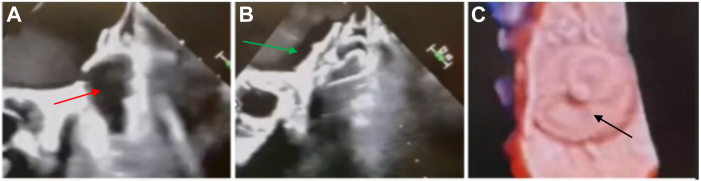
Case :1 incomplete surgical LAA closure successfully occluded with Amplatzer Amulet (22 mm). A: Preprocedural TEE shows incomplete surgical LAA closure with a narrow residual neck and persistent flow from the left atrium into the LAA remnant (*red arrow*). B: After transseptal access and delivery of a 22 mm Amplatzer Amulet, the lobe is seated in the remnant and the disk (*green arrow*) covers the atrial opening. Color Doppler demonstrated no peridevice leak, confirming immediate occlusion. C: 3-dimensional TEE confirms a well-seated device (*black arrow*) with complete coverage of the residual neck and stable device position. LAA = left atrial appendage; TEE = transesophageal echocardiogram.
